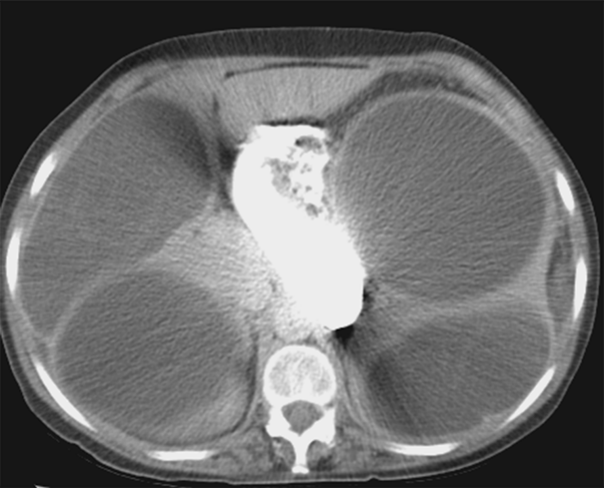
Computed tomography showing hydatid cysts of the liver and spleen.
